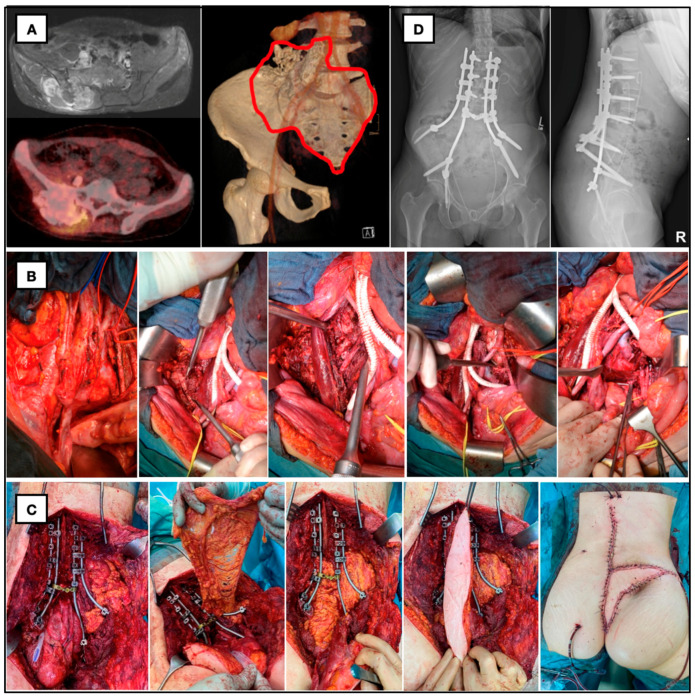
Twenty-eight-year-old female patient with giant ilio-lumbosacral osteosarcoma. (A) Preop. MRI, PET and CT with planned resection margins. (B) Anterior approach with aortic bifurcation, vascular replacement of vena cava, osteotomy of the right iliac wing and discectomy of L4/L5. (C) En bloc resection of os sacrum and right iliac wing, spinopelvic fixation with double-rod construction, preparation of omentum majus for death space filling and defect reconstruction with VRAM-flap. (D) Postop. X-ray with iliolumbar/spinopelvic fixation.
